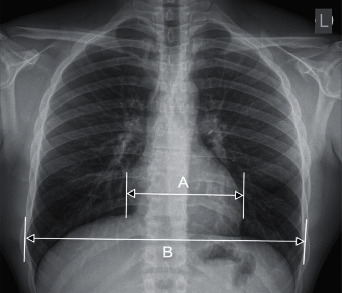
The method of measuring CTR on a chest radiograph in the PA projection. CTR = A/B.
